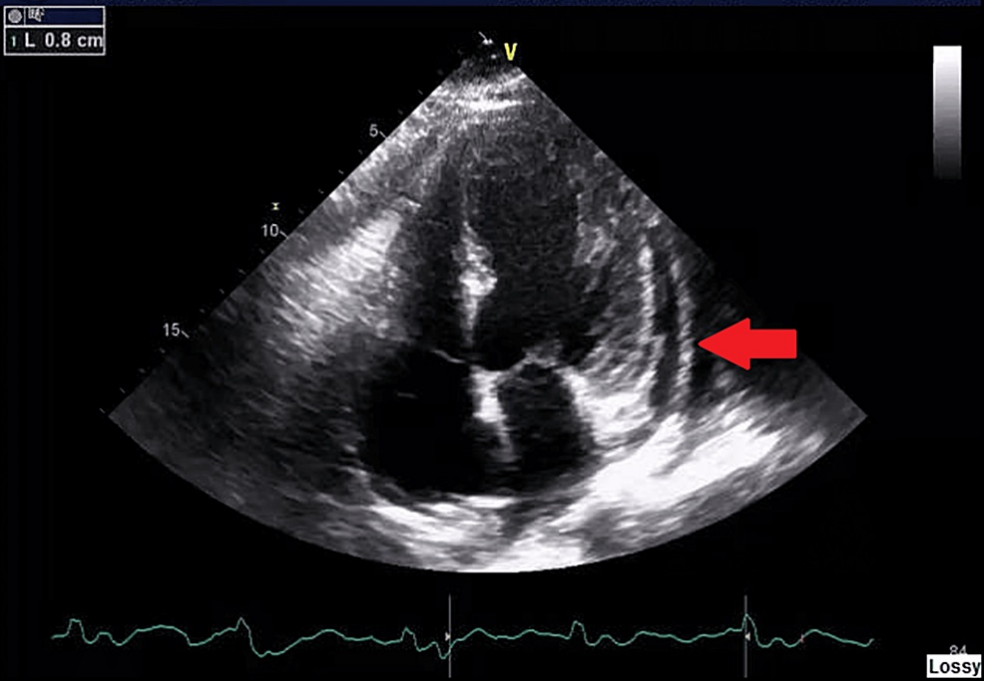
A4C view of 2D TTE showing a small pericardial effusion. A4C: Apical four-chamber, 2D: Two dimension, TTE: Transthoracic echocardiogram
